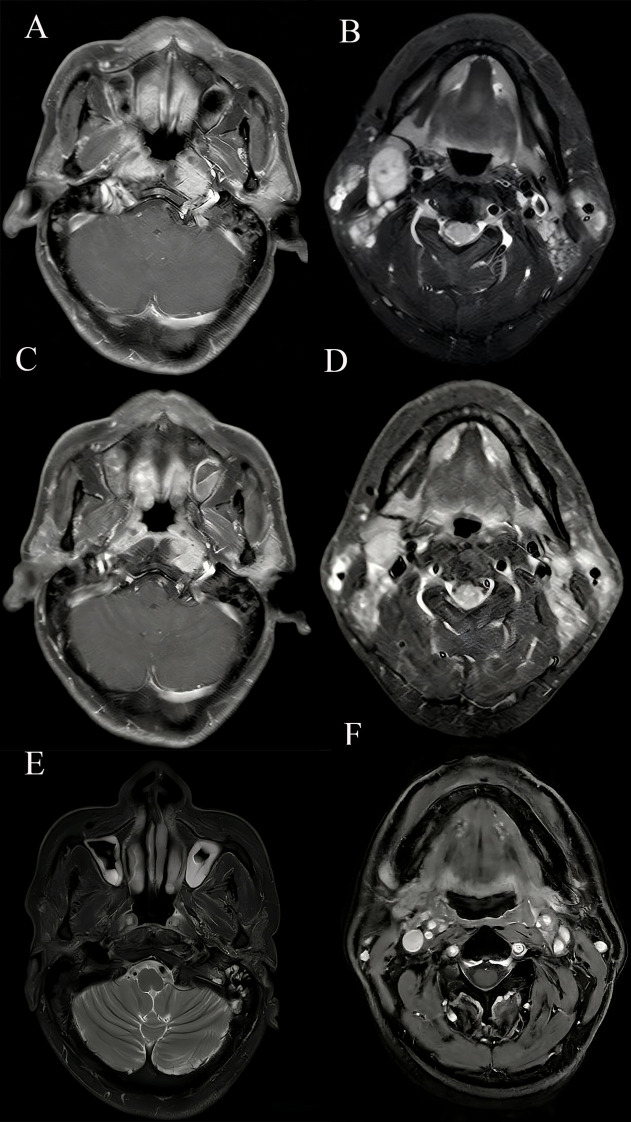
Computed tomography (CT) images obtained during the course of treatment. (A, B) CT images of the lesion at initial presentation: nasopharyngeal lesion (A) and lymph node (B). (C, D) CT images after three cycles of chemotherapy. (E, F) Current CT findings on January 16, 2026, showing no evidence of tumor recurrence.
